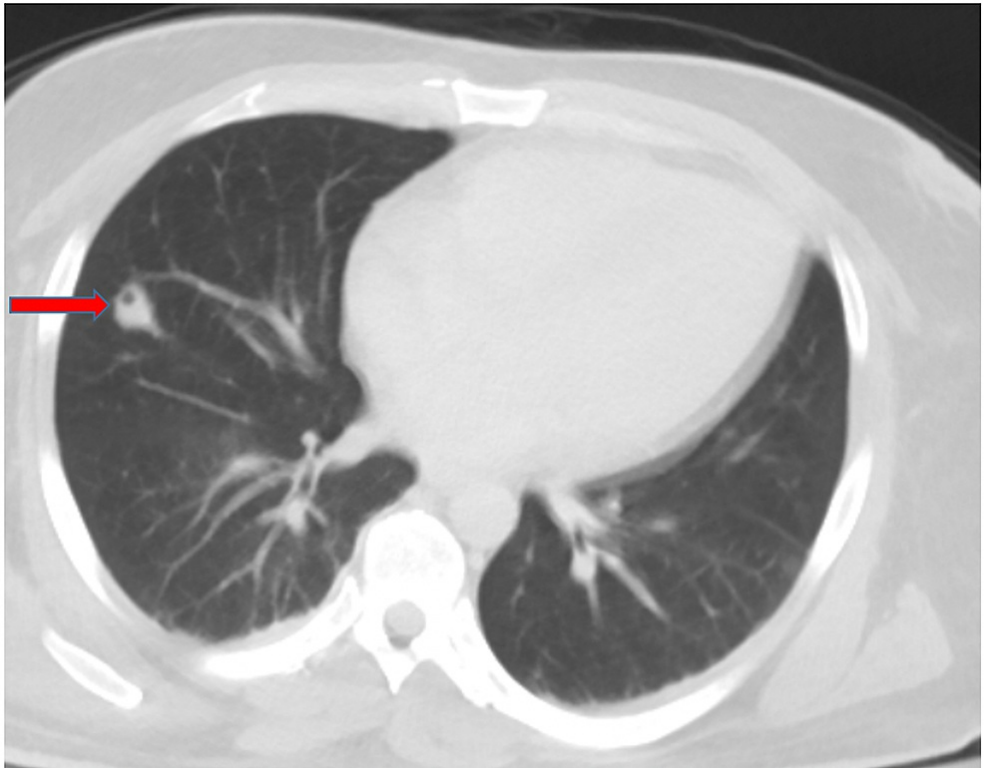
CT pulmonary angiography with septic embolus in the right lung as shown by the red arrow.
